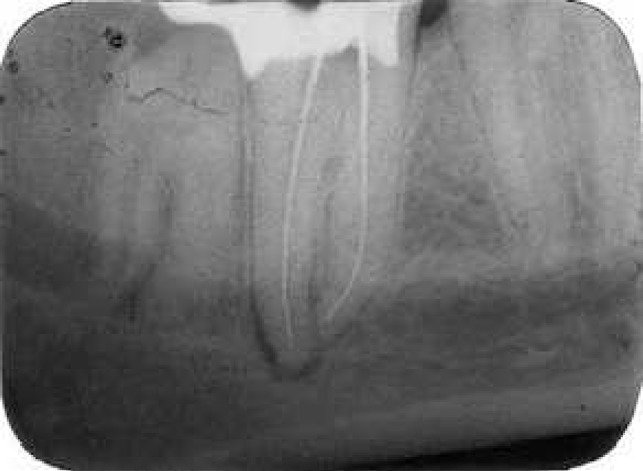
The working length measurement radiograph showed three files in three separated roots
